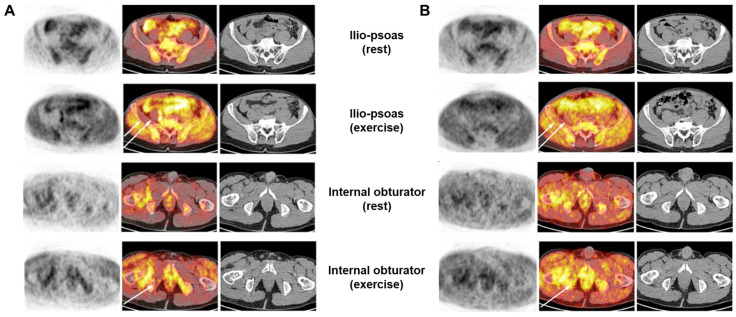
PET/CT with [^11^C]acetate was conducted at three weeks (A) and three months (B) after right hip arthroplasty using the posterior “Kocher Langerhans” approach. The images depict the iliopsoas and obturator muscles, with the upper and lower rows displaying resting and after-exercise PET, respectively. In each row, from left to right, the images represent PET alone, PET/CT superposition, and low-dose CT alone. The notable surge in [^11^C]acetate uptake during exercise was similarly observed on the unaffected side of the body in the iliac, psoas, and internal obturator muscles. Conversely, on the operated side, these muscles (indicated by arrows) exhibited similar activity levels during rest and exercise at the 3-week mark. The postsurgical [^11^C]acetate PET hyperactivity extending into the external obturator muscle on the right side, directly related to the surgical approach, remained stable at rest and exercise. The iliopsoas and obturator muscles affected by surgery ((B), indicated by arrows) show almost complete recovery. [^11^C]acetate uptake from surgical sites increased with exercise, similar to [^11^C]acetate uptake from healthy body sites. CT, computed tomography; PET, positron emission tomography. Reproduced from [36], copyright © 2011 Molecular Imaging and Biology.
